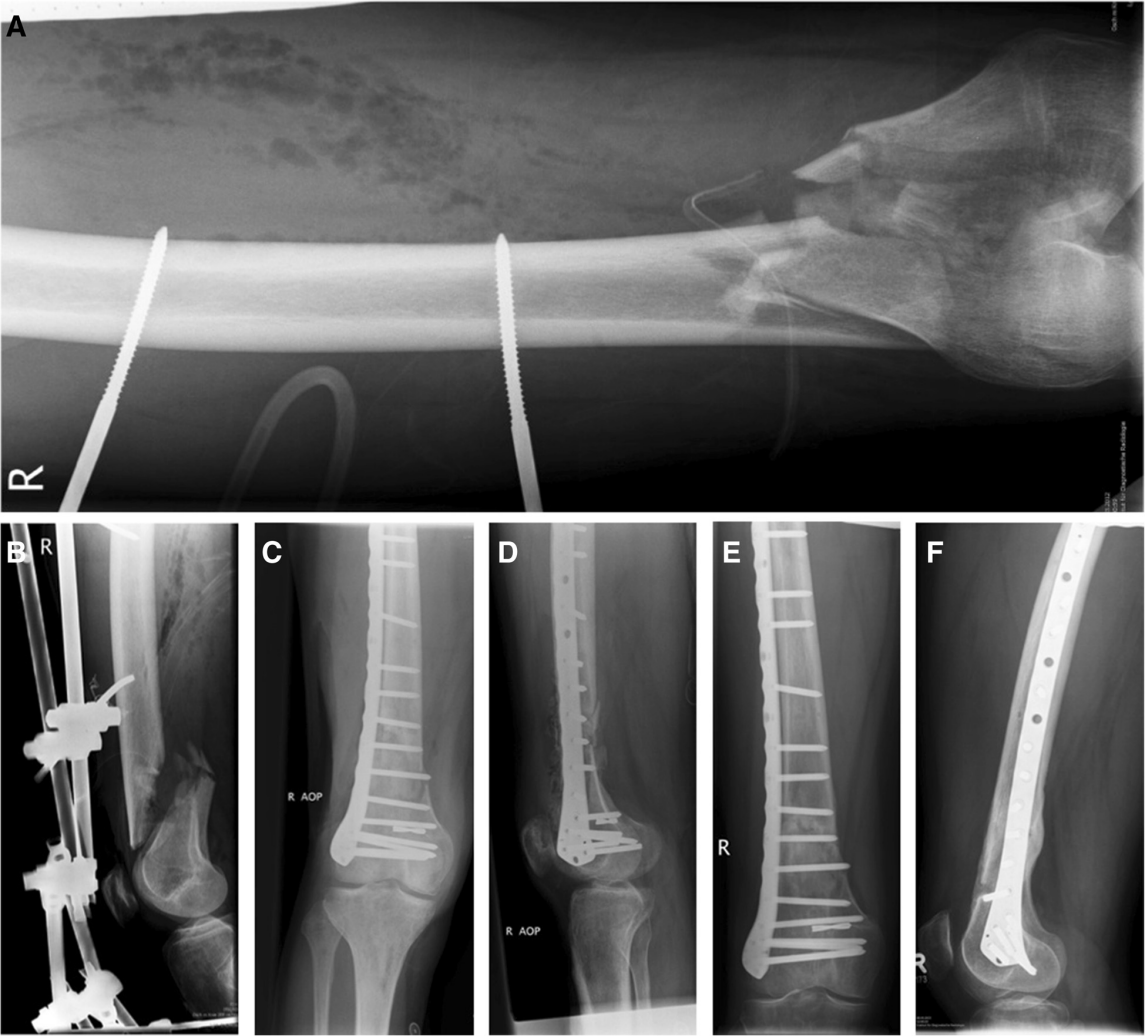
Treatment and follow-up of a distal femoral fracture. (A) Preoperative radiographic AP view of a distal femur fracture with external fixation. (B) The lateral view shows the sagittal alignment of the fragments. (C,D) Postoperative radiographs confirm reduction quality and implant position. (E,F) Callus formation and cortical continuity demonstrate ongoing fracture healing.
